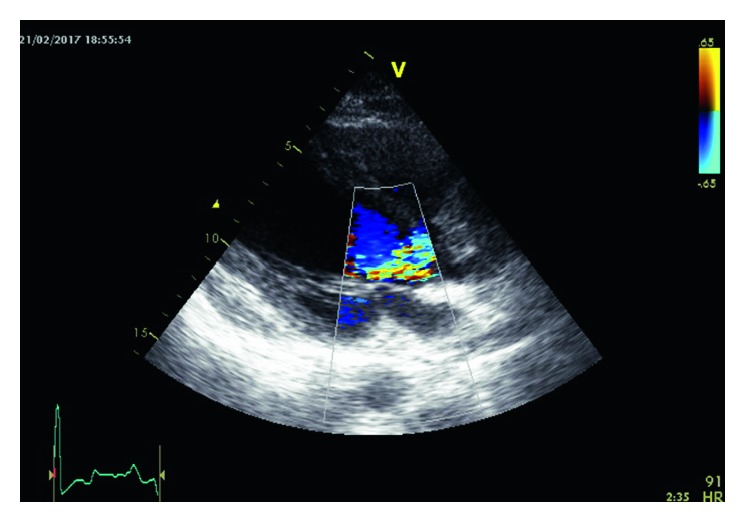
Doppler echocardiography and colour flow mapping revealed moderate-to-severe (3/4) aortic valve failure.
